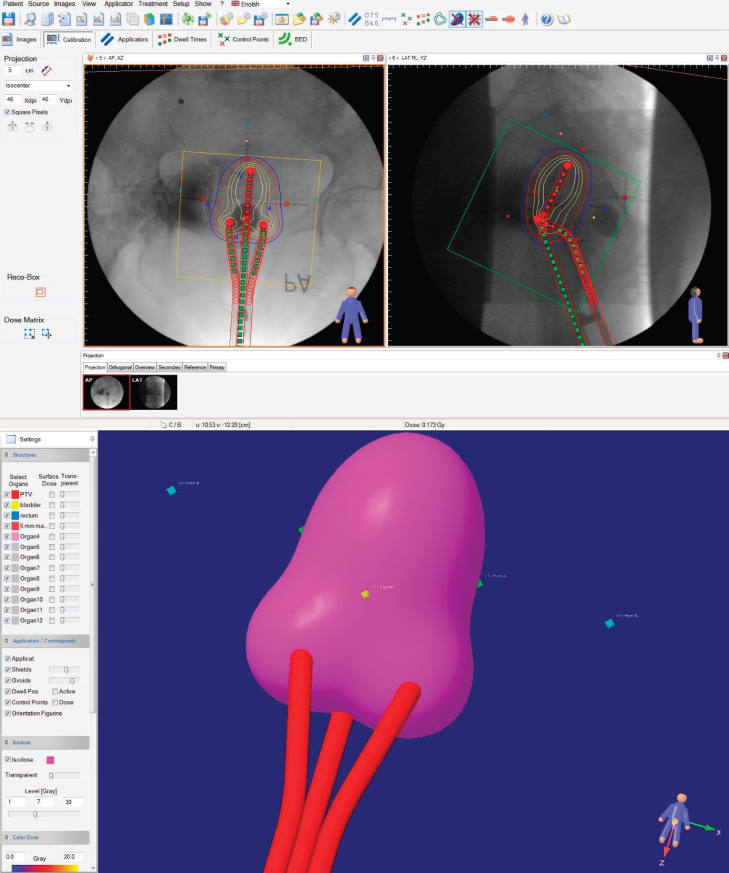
Development of the treatment plan with X-ray images in the HDR plus TPS. The applicators and point positions are saved in a cluster for exporting to the image sequence plan.
